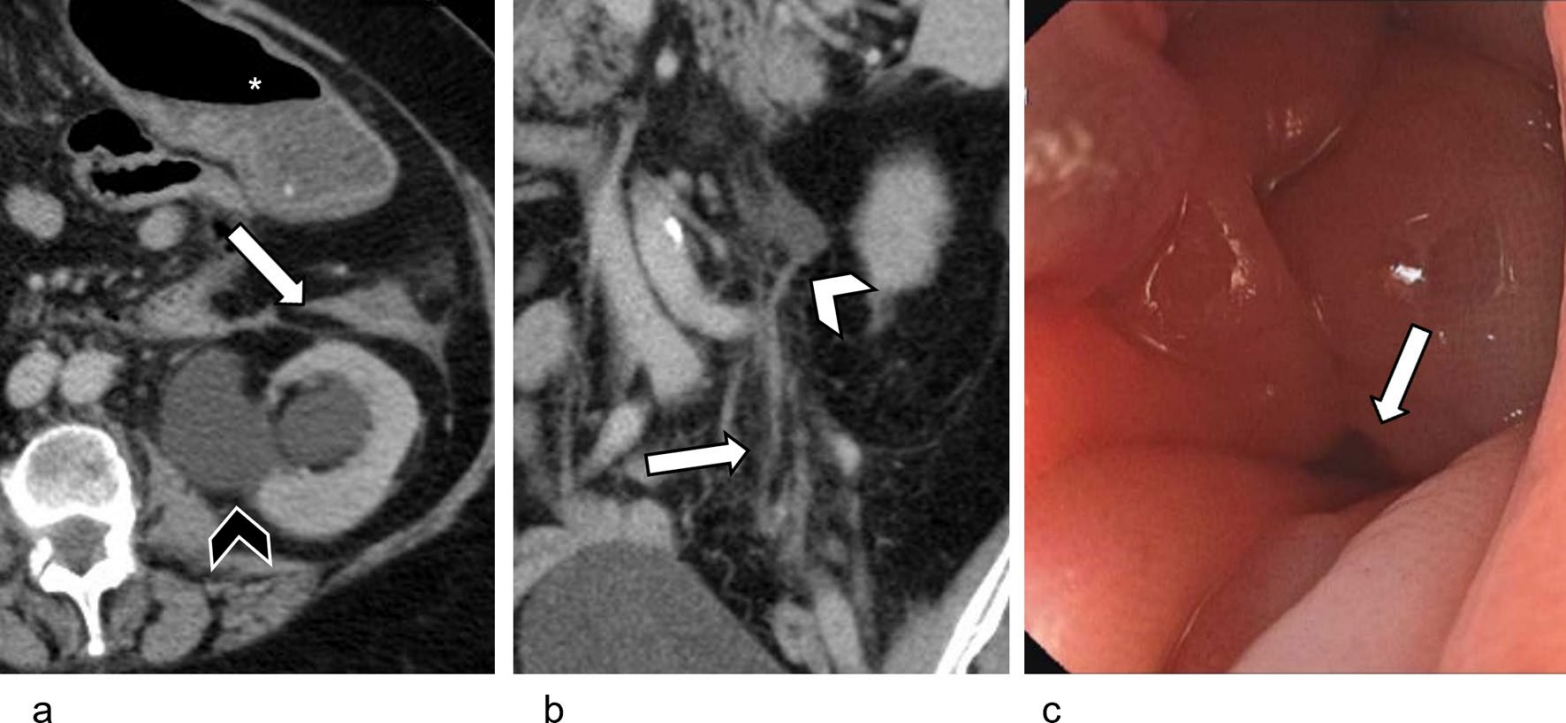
Metastatic retroperitoneal involvement in an 80-year-old female, who presented with symptoms of bowel obstruction. She had declined surgical treatment for Stage 2B ILC a year earlier and was on letrozole. a Axial contrast-enhanced CT image shows a stricture of the descending colon, with a tethered, tapered appearance at its medial aspect (white arrow). The upstream transverse colon (*) is dilated. Left-sided hydronephrosis is also seen (black arrowhead). b Coronal contrast-enhanced CT image shows tiny metastatic nodules and stranding in the left side of the retroperitoneum (white arrow). The transition points of the left hydronephrosis (white arrowhead) and descending colon (not pictured) are within this nodular area. c Colonoscopy confirms a stricture of the descending colon (white arrow). The mucosa appeared normal on colonoscopy, and this was initially thought to represent a benign stricture. Subsequent open laparotomy confirmed disseminated metastatic disease in the retroperitoneum
